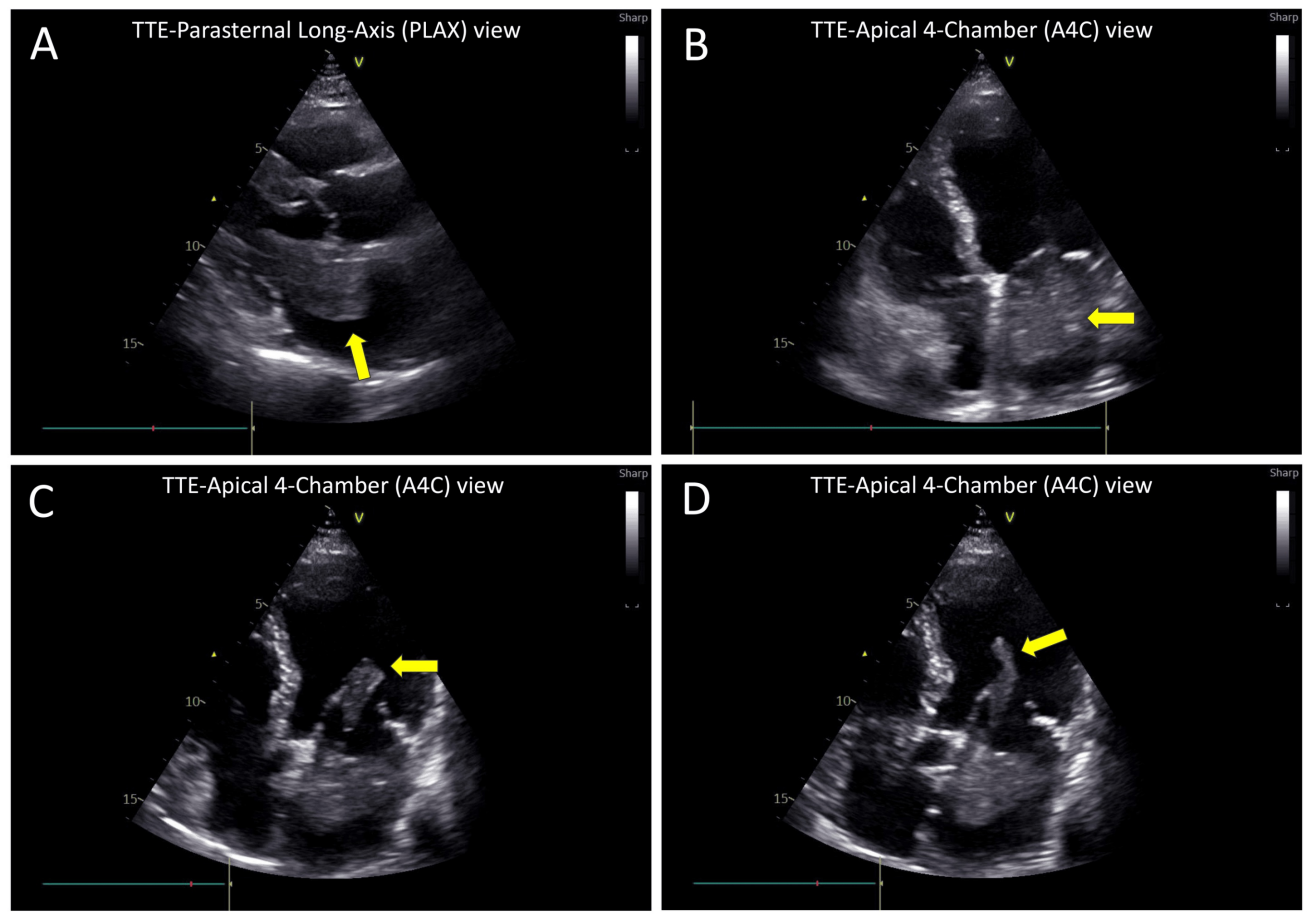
Transthoracic echocardiography (TTE) TTE showing a large mass in the left atrium (LA) abutting the mitral valve (MV). Panels A-D: (A) Parasternal long-axis view, (B) Apical four-chamber view in systole showing a round mass in the LA attached to the interatrial septum, positioned near the MV, (C) Apical 4-chamber view in early diastole showing finger-like pedunculation attached to the LA mass, prolapsing through the MV, almost completely obstructing left ventricular inflow. (D) Apical four-chamber view in late diastole showing pedunculation projecting further into the left ventricle with partial obstruction of inflow.
